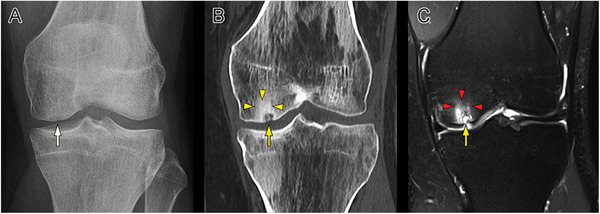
Radiographic images of a 30‐year‐old male patient with osteochondritis dissecans (OCD) (A) at the left medial femoral condyle initially treated with the subchondral drilling and subsequent symptomatic subchondral bone cyst formation (B, C) at 60 months postoperatively. The white (A), yellow (B), and red arrows (C) indicate the subchondral bone cyst. The yellow arrowheads (B) designate the area of the OCD lesion surrounding the subchondral bone cyst in the CT image. The red arrowheads (C) denote the high signal intensity of the diffuse bone marrow edema (BME) around the cyst in the T2‐weighted MRI image
